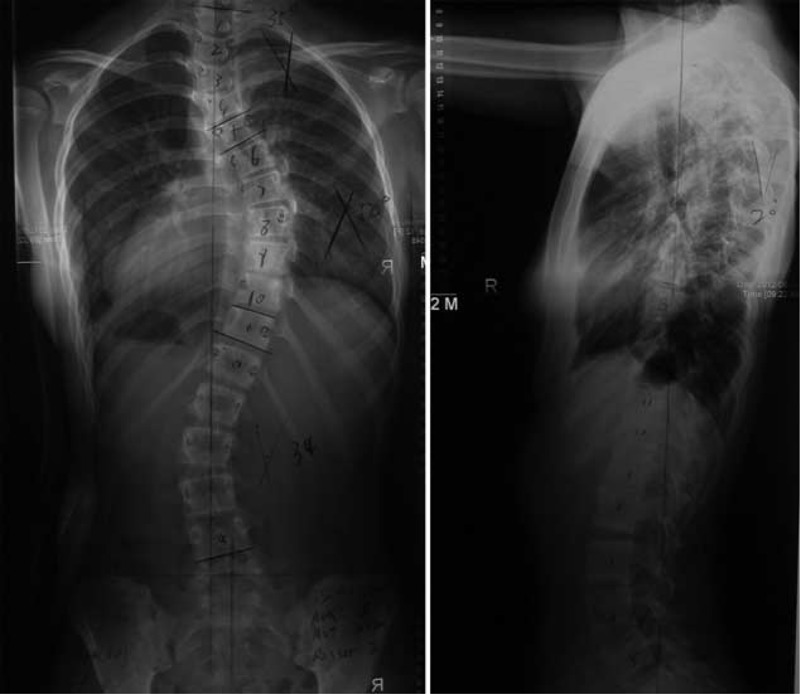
Standing anteroposterior and lateral radiographs of preoperation.
